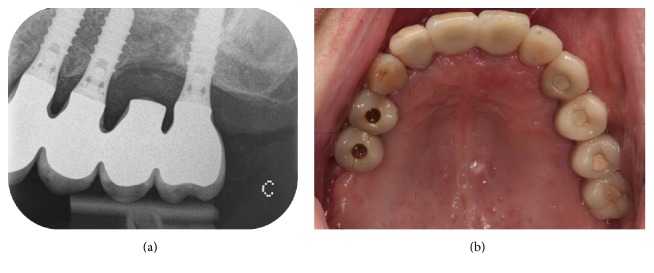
The implant 25 was removed and the bridge was rescrewed. The patient underwent antibiotic treatment. (a) Periapical radiograph and (b) clinical view.
